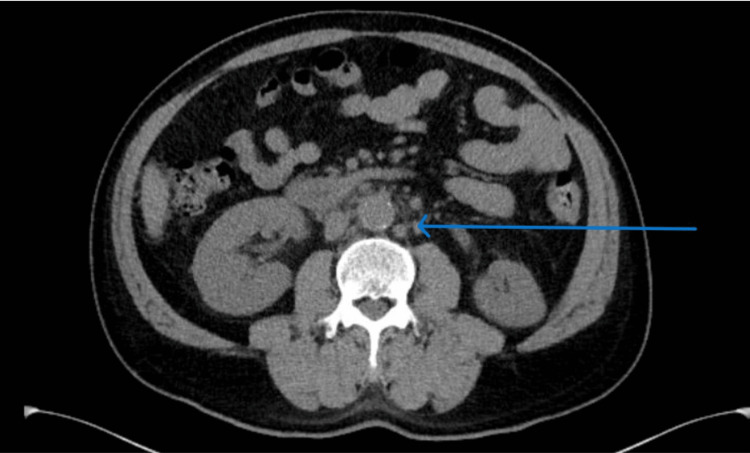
CT scan of the abdomen and pelvis with mesenteric lymphadenopathy. CT: computed tomography.
